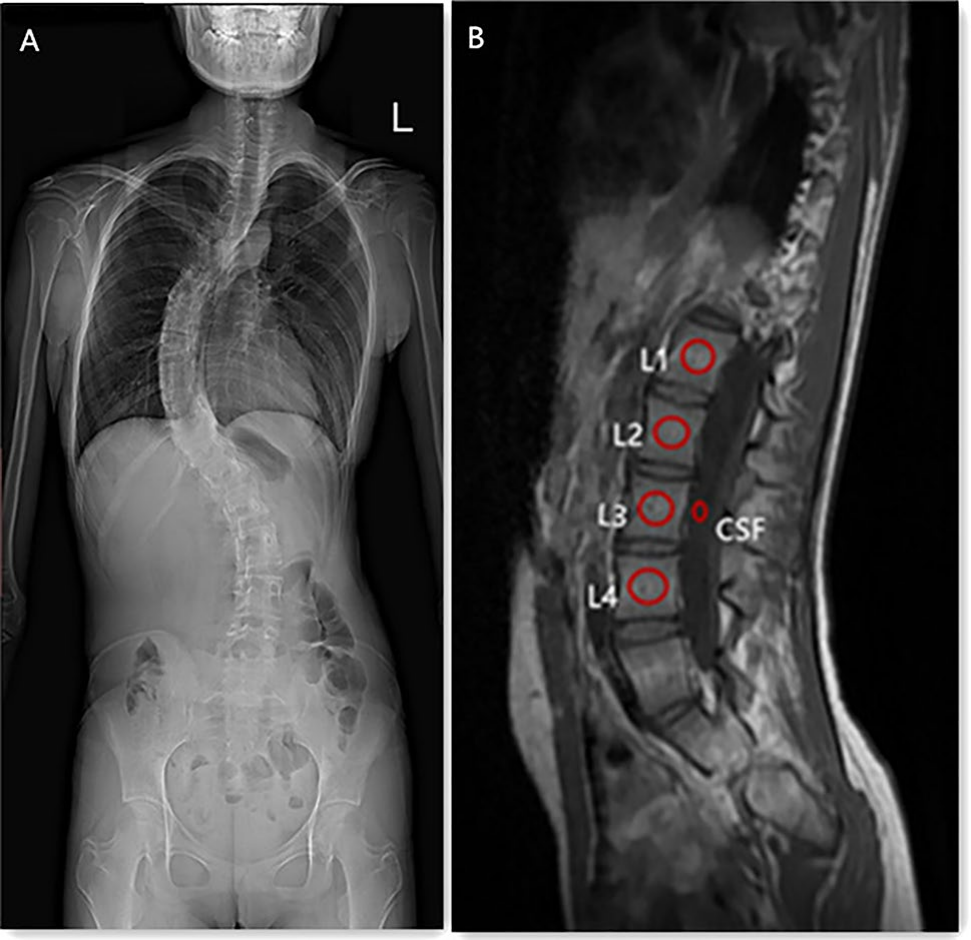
(A) a standing full-length posteroanterior plain radiography of an AIS patient with aged 16 years. (B) The signal intensity of regions of interest (circles) utilized for the computation of L1-L4 vertebral bone quality (VBQ) score on sagittal non-contrast-enhanced T1-weighted MRI.
